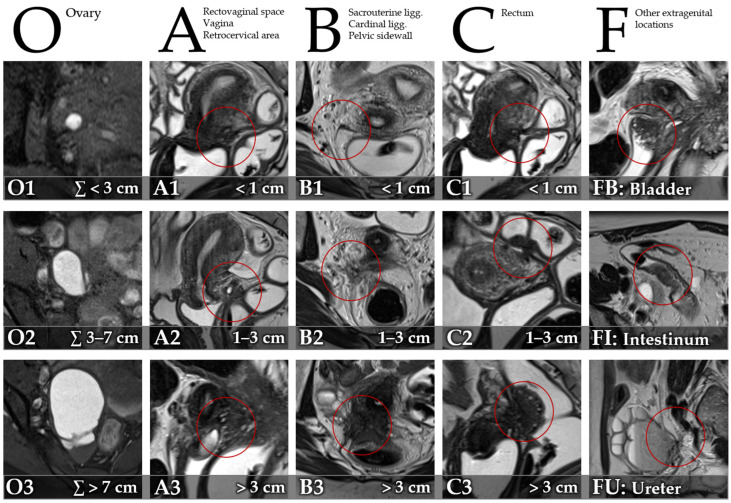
Description of the Enzian classification of endometriosis on MRI for compartments/organs O (T1-weighted FSE [fast spin echo] with fat suppression, axial), A (T2-weighted FSE, sagittal), B (T2-weighted FSE, axial), C (T2-weighted FSE, sagittal), FB (T2-weighted FSE, sagittal), FI (T2-weighted FSE, axial), and FU (T2-weighted FSE, sagittal), adapted from Keckstein et al. [15].
